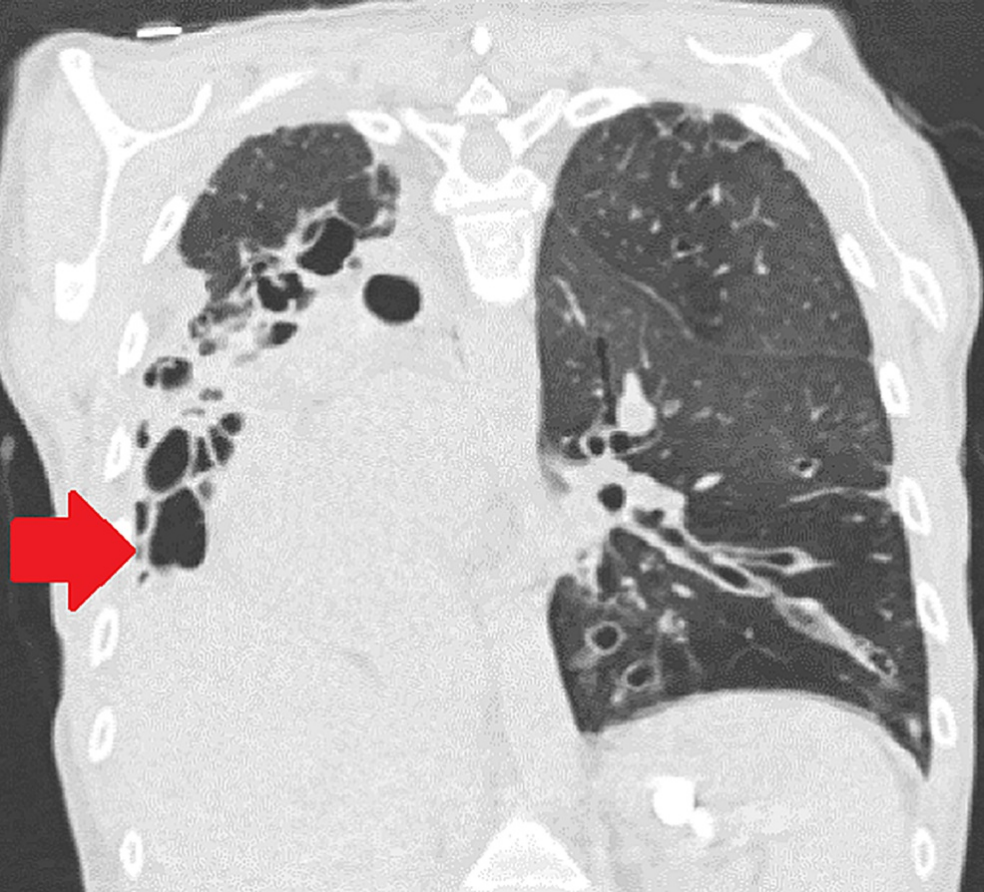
CT chest coronal view shows lower lobe predominant bronchiectasis, heart deviated to the right with extreme right lung destruction (red arrow)
